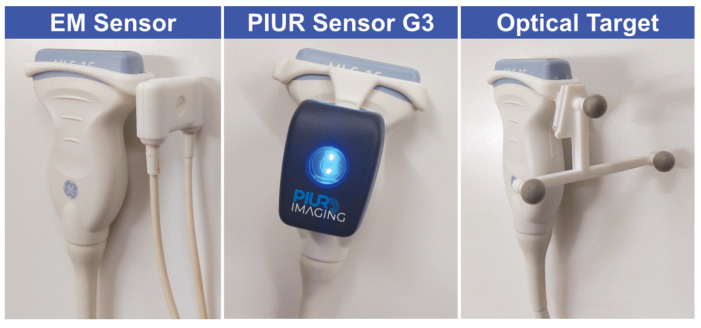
Add-ons for ultrasound probe in free-hand tracked ultrasound technologies.
